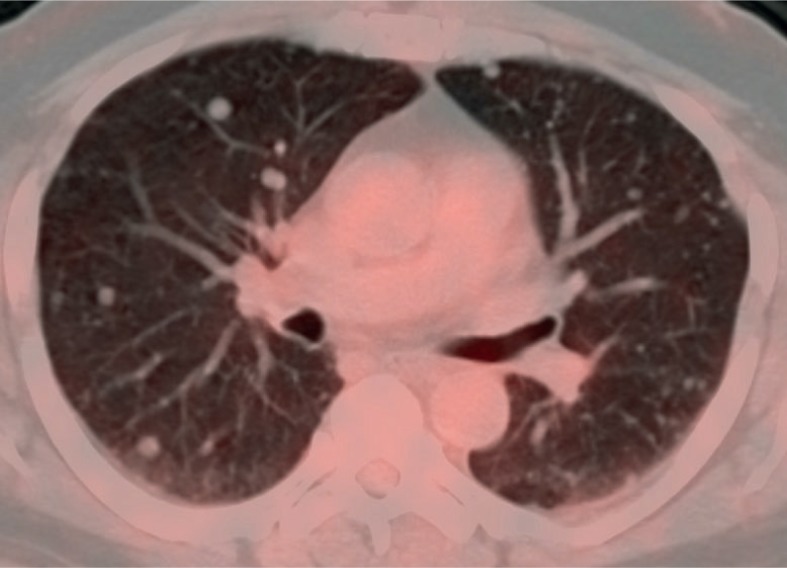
FDG-PET/CT images show no significant FDG uptake in numerous lung metastases.
